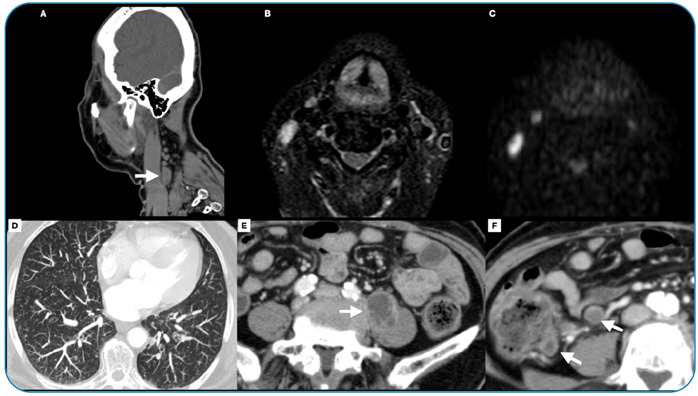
CT and MRI of the neck showing lymph node enlargement in the right level V (white arrow in A, B, and C) and a CT of the chest showing the presence of numerous small diffuse peripheral nodules (D). A CT of the abdomen also revealed an abscess in the left iliopsoas muscle (E) and necrotic lymph nodes (F), demonstrated with a white arrow.
